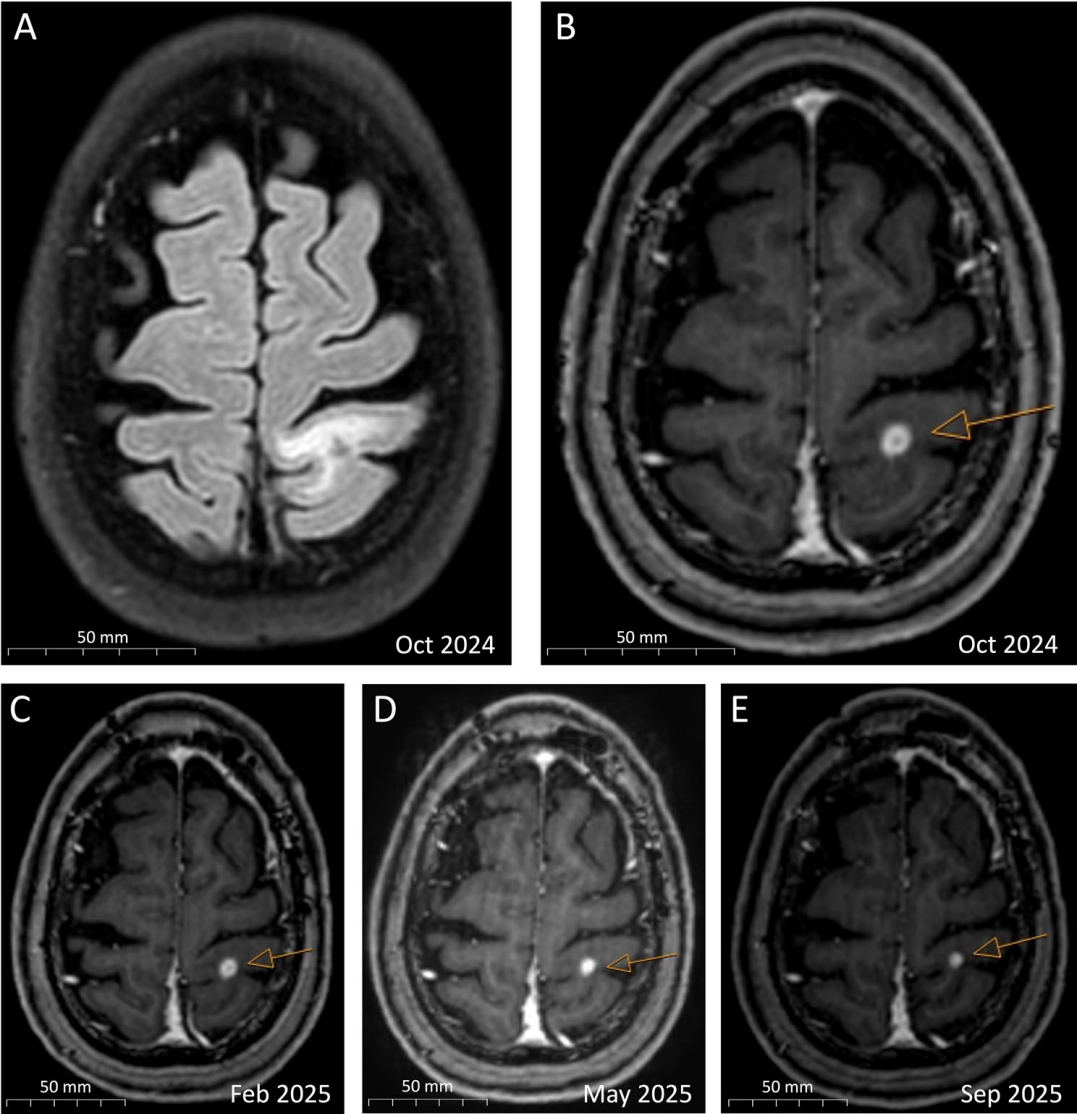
cMRI scans showing a ring-enhancing lesion (orange arrows) with surrounding edema in the left postcentral gyrus, decreasing in size from 8 mm to 4 mm within a 12-month time span. A: cMRI scan, axial plane, FLAIR. B–E: cMRI scans, T1 weighted, axial plane, contrast enhanced
